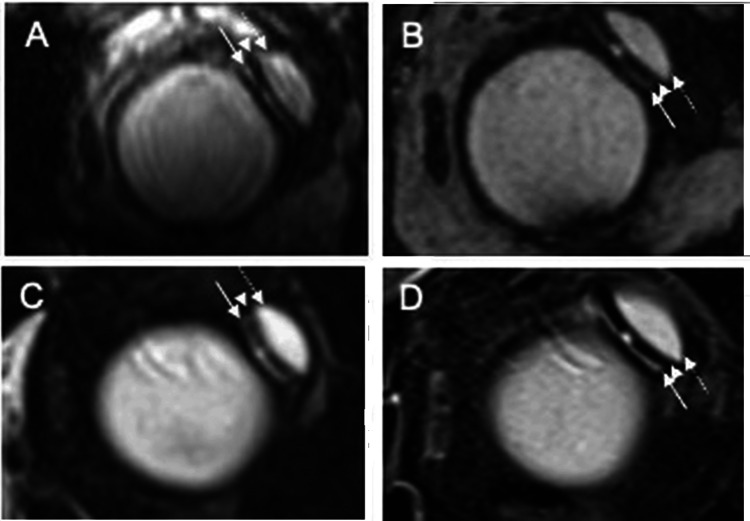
Long-term preservation of double-layered bleb morphology on orbital MRI (A,C) Images from the same operated eye (Eye 1). (B,D) Images from another operated eye (Eye 2). (A,B) T2-weighted images obtained at approximately nine months postoperatively. (C,D) Corresponding images obtained at approximately six years postoperatively. A hyperintense fluid signal consistent with aqueous fluid forms a double-layered bleb on the scleral side (solid arrow) and conjunctival side (dashed arrow) of the endplate. The endplate is identified as a low-intensity structure between the bilayered bleb (arrowheads) MRI: magnetic resonance imaging
